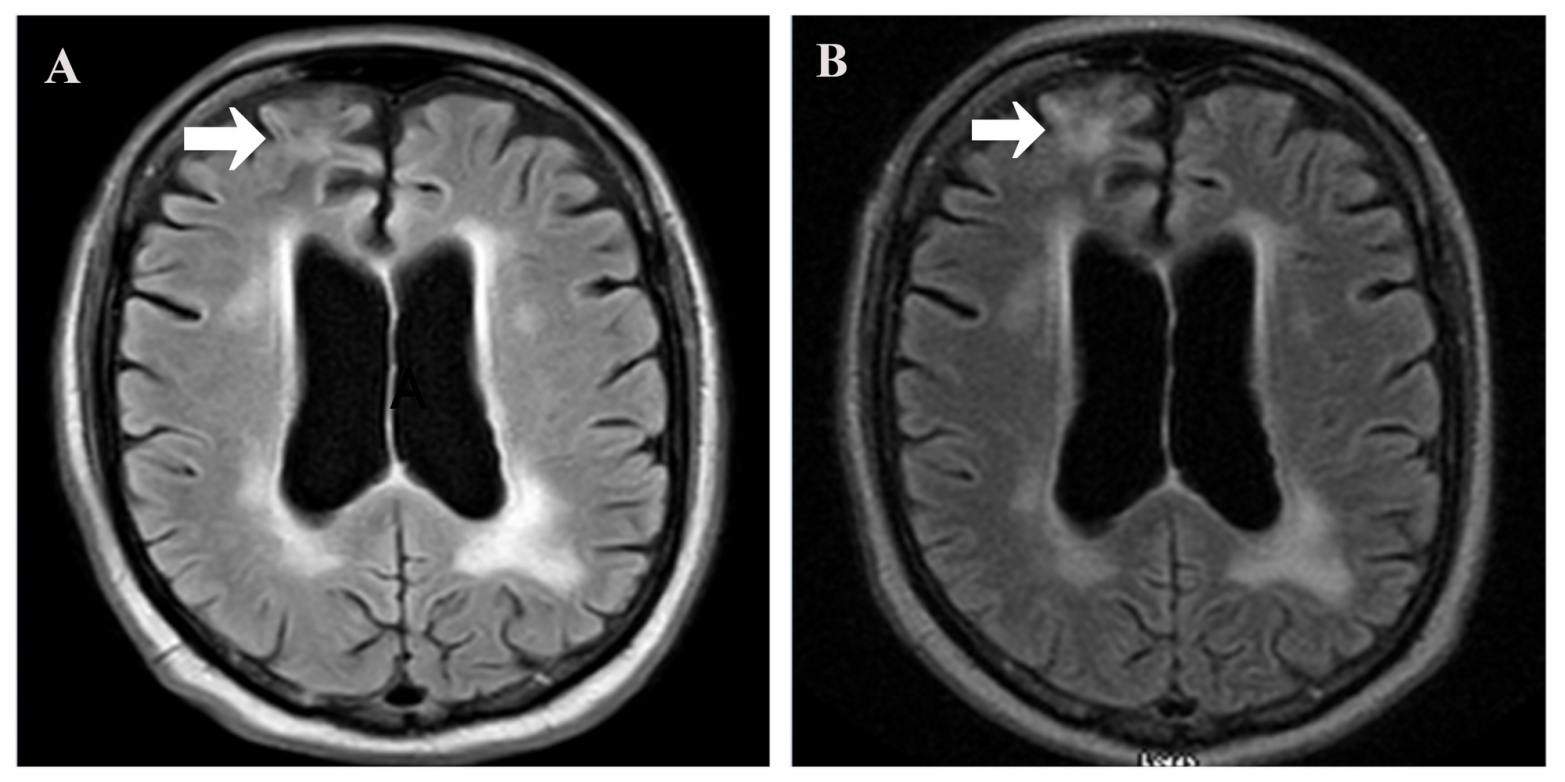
(A) Magnetic resonance imaging (MRI) of the brain with/without contrast showing disappearance of lesion in the right frontal lobe and stable lesions in other cerebral lobes as compared to MRI with/without contrast (B) done after six months of nivolumab therapy.
